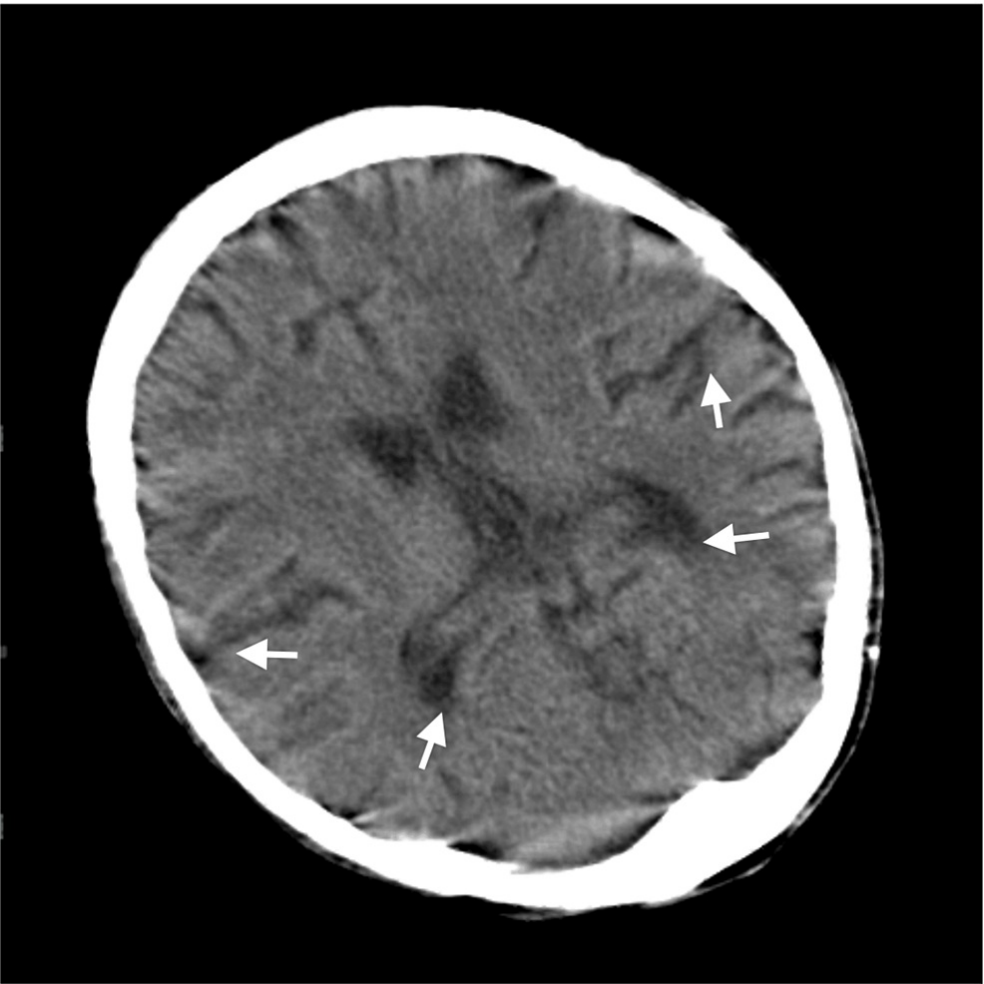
Brain CT on admission showed mild brain atrophy, suspected to be caused by long-term malnutrition
